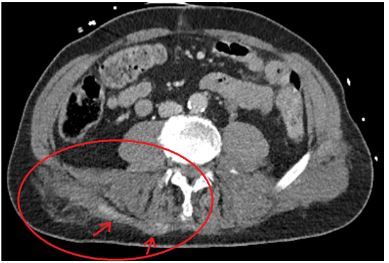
Computed tomography scan of the abdomen on arrival at the emergency department showing the early signs of a Morel-Lavallée lesion in the lumbar region. The oval shows the main lesion. The arrows indicate the contrast extravasation.
